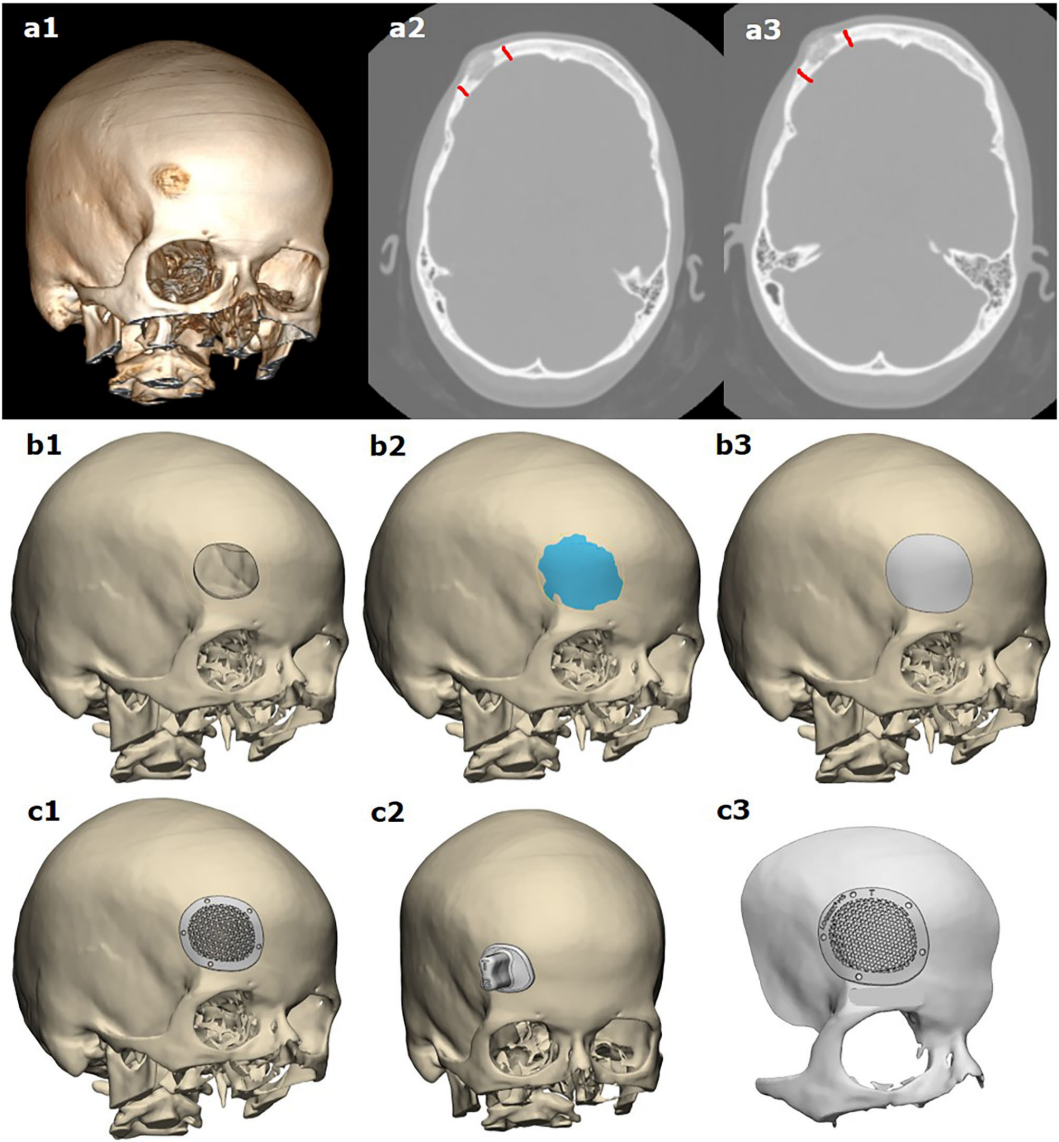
Modeling of template-assisted resection of bone tumor, following cranioplasty based on virtual planning of the resection margins: (a1–a3) based on CT scans, planning of resection margins made by the surgeon; (b1–b3) 3D modeling of tumor resection and the anatomical shape of the resected part of the bone; (c1, c2) 3D modeling of the implant and resection template; (c3) a pre-operative planning template.
